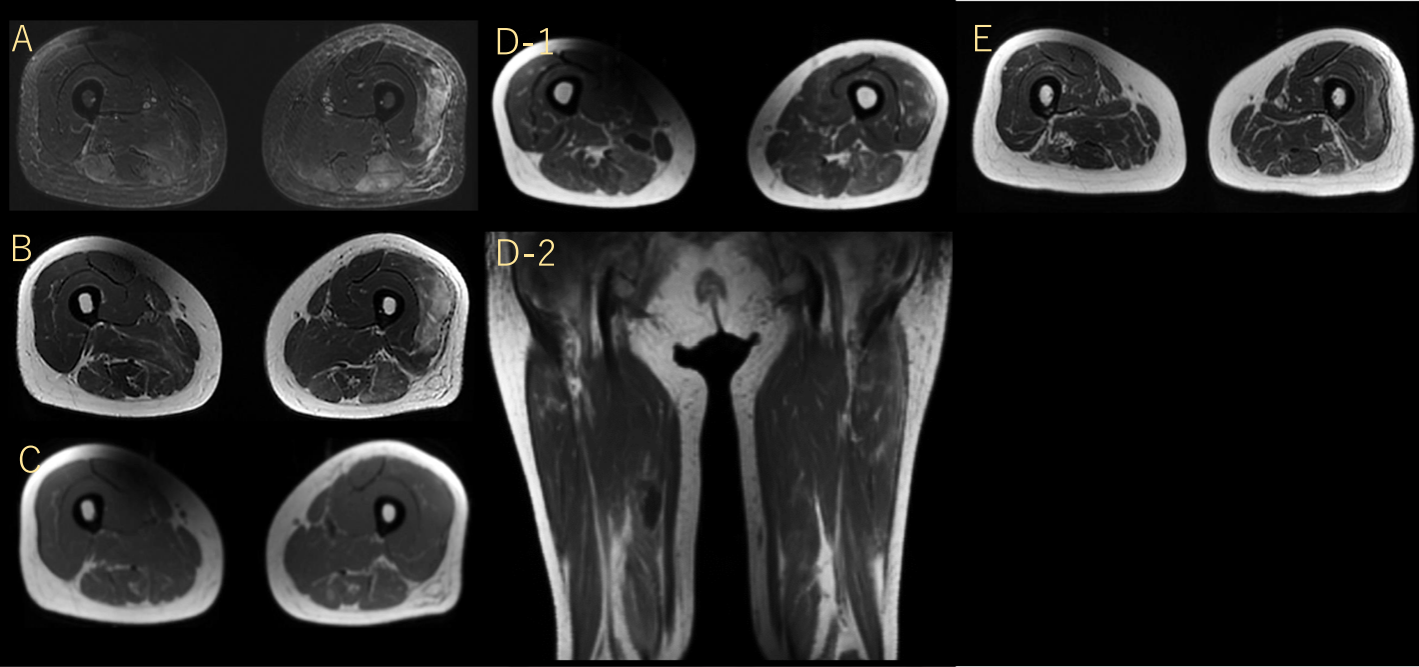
Femoral MRI images before A–D and after E muscle biopsy. STIR image (A). T2-weighted image (B). T1-weighted image (C). Gadolinium-enhanced image (axial; D-1, coronal; D-2, respectively). Follow up MRI on day 33 (T2-weighted image) (E). MRI: magnetic resonance imaging, STIR: short T1 inversion recovery
